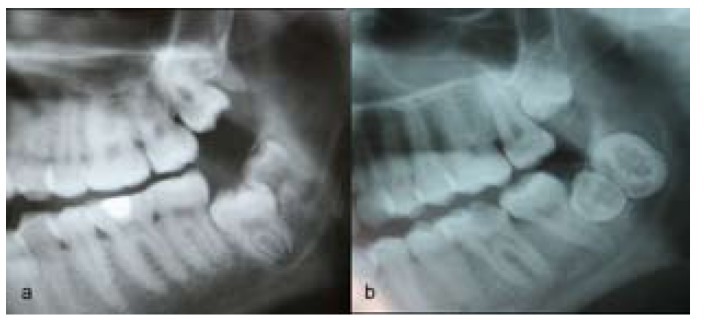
Radiographic appearance of hypomineralized distomolar tooth (a), an example of macrodontic distomolar tooth (b).
